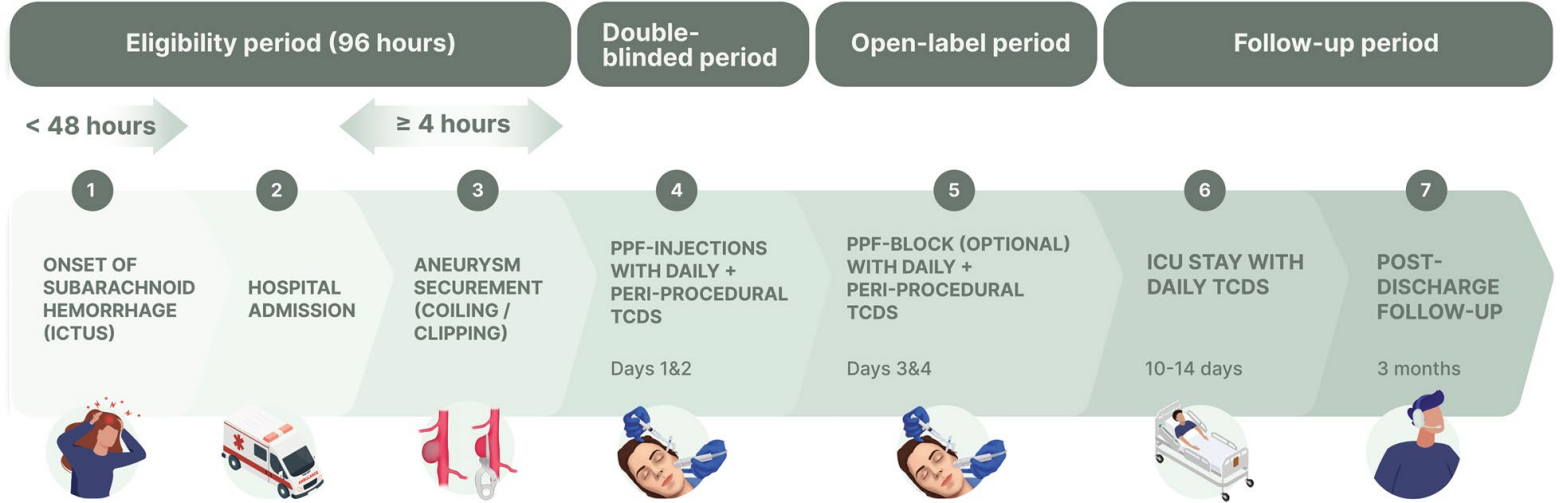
Study timeline depicting the participant-facing events of BLOCK-SAH. ICU intensive care unit, TCD transcranial Doppler
